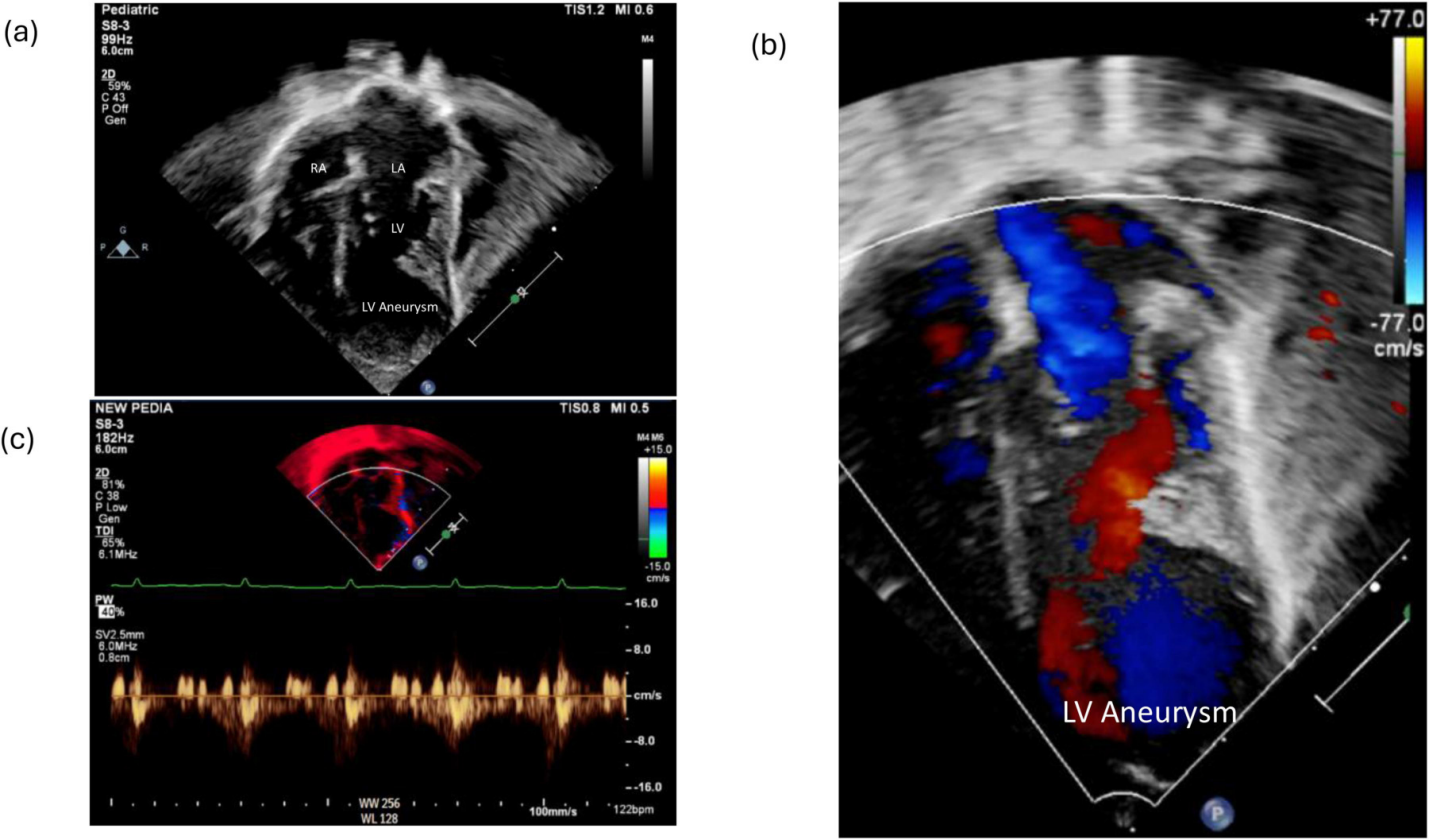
(a) Echocardiography showing a four-chamber view showing a left ventricular apical aneurysm. (b) Echocardiography showing a color Doppler two-chamber view showing a left ventricular apical aneurysm with swirling flow within the aneurysm. (c) Tissue Doppler echocardiography showing a left ventricular apical aneurysm with reduced lateral wall velocity. RA, right atrium; LA, left atrium; LV, left ventricle.
